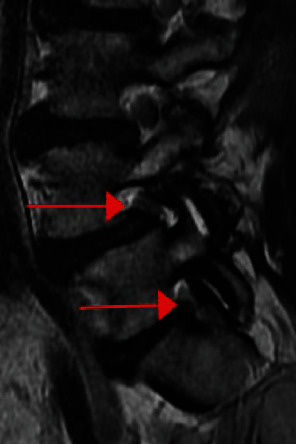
Preoperative T2 sagittal MRI demonstrating spondylolisthesis at L4-5 and foraminal stenosis at both L4-5 and L5-S1.
